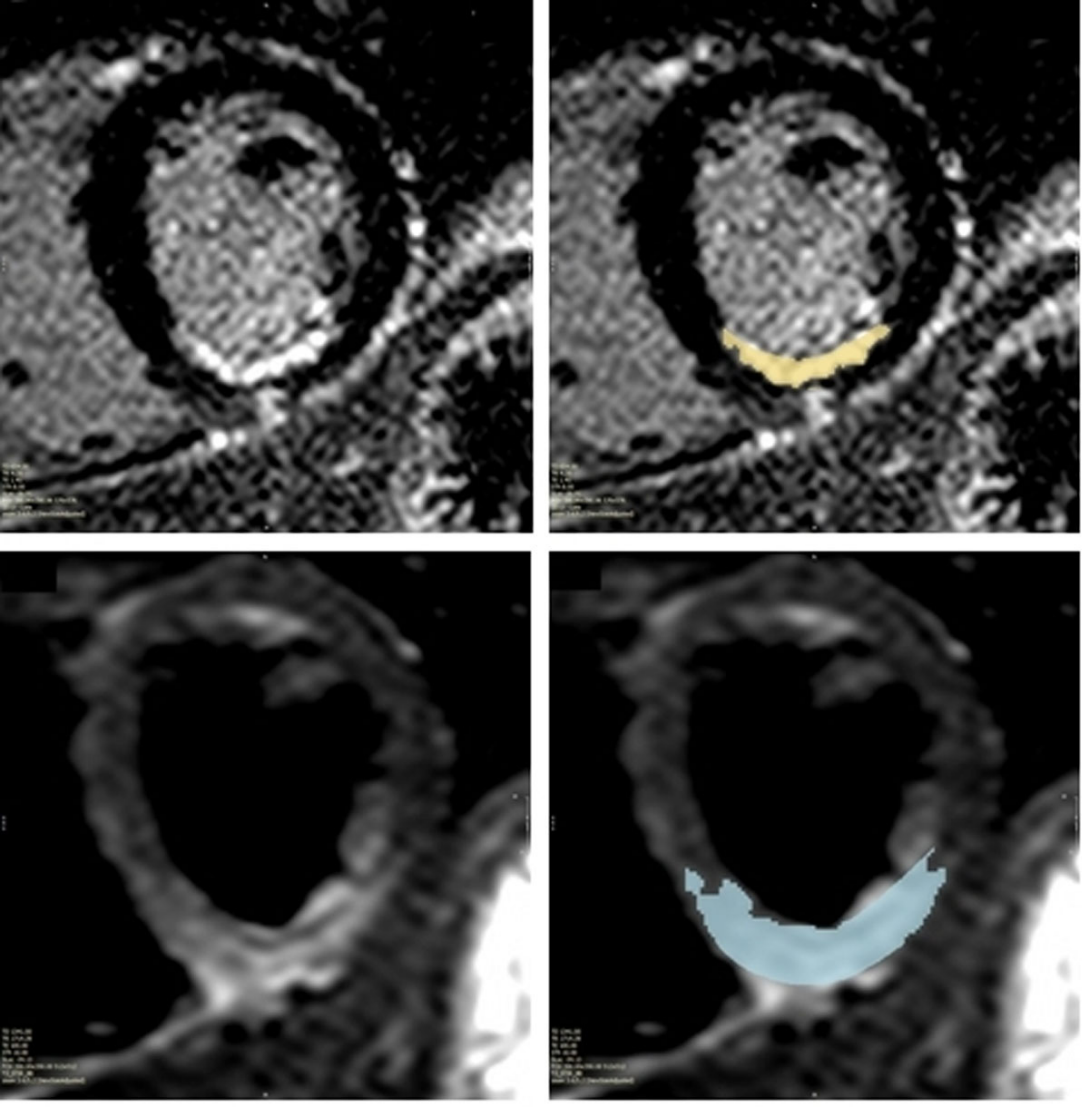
Quantification of myocardial necrosis (upper) and salvage (lower) on MRI following PPCI in a current smoker.
